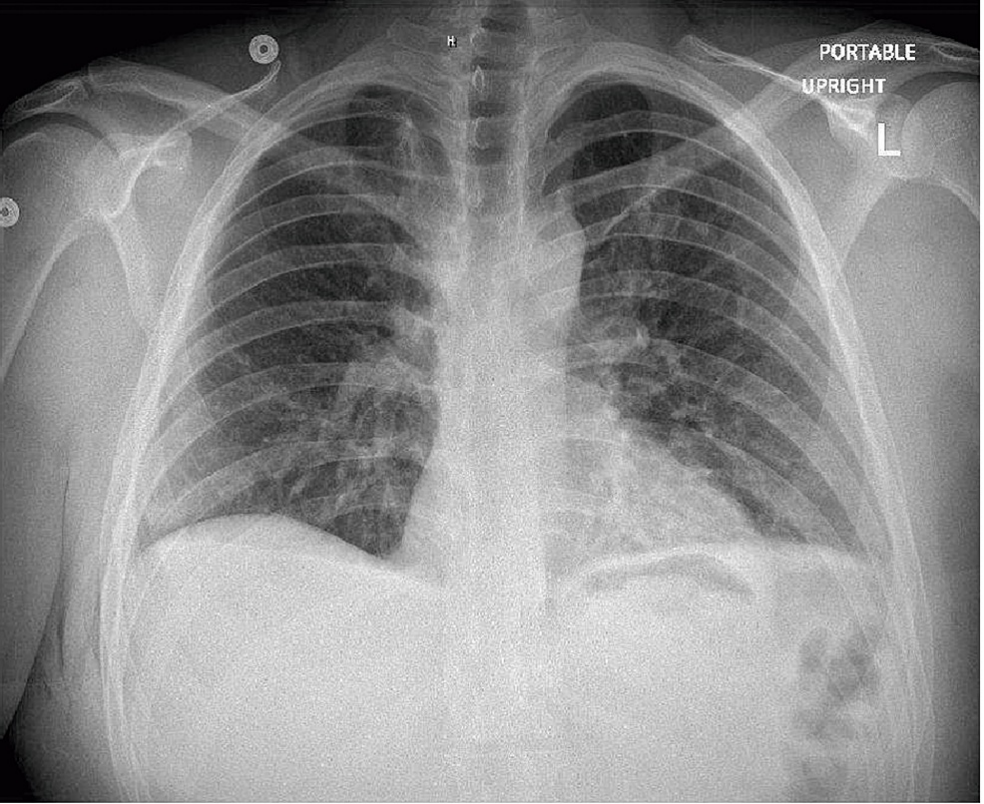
CXR on the day of discharge showing resolution of pneumothorax CXR, chest X-ray
